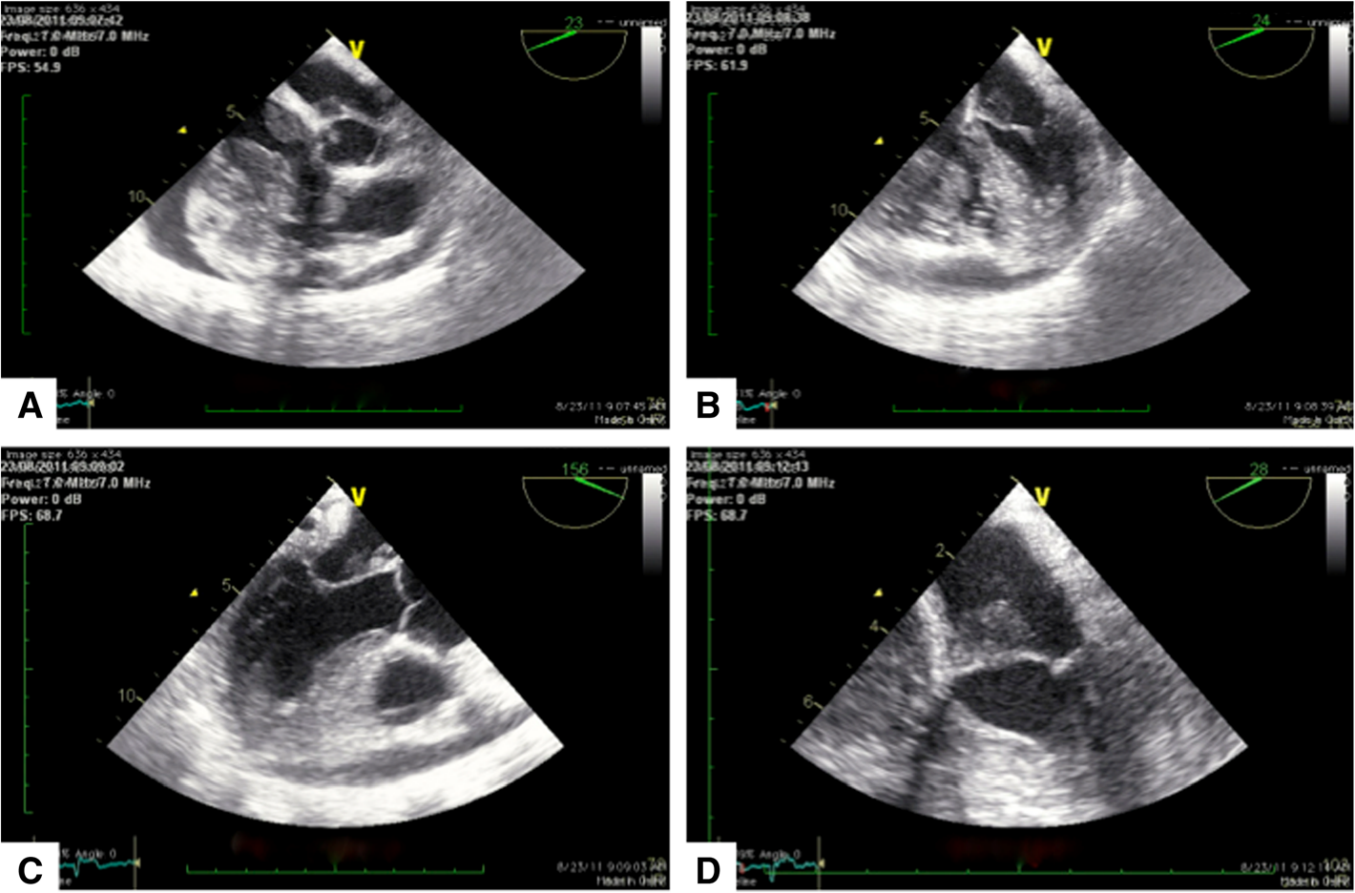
Repeat transesophageal echocardiography (TEE) study. A 5-chamber view. Tumor formation in both left and right sided cardiac structures, mitral and tricuspid valves are affected. Of note, both, the right atrium and the right ventricle are significantly obstructed. B Mid-esophageal 4-chamber view confirming extensive tumor formation and showing significant involvement of the mitral valve leaflets. Both, the right atrium and the right ventricle are severely obstructed. C Mid esophageal long axis confirming mitral valve involvement. D 4-chamber view, with cut apex. Tumor formation on mitral valve, however mitral valve apparatus does not appear to be diffusely infiltrated or misconfigured.
